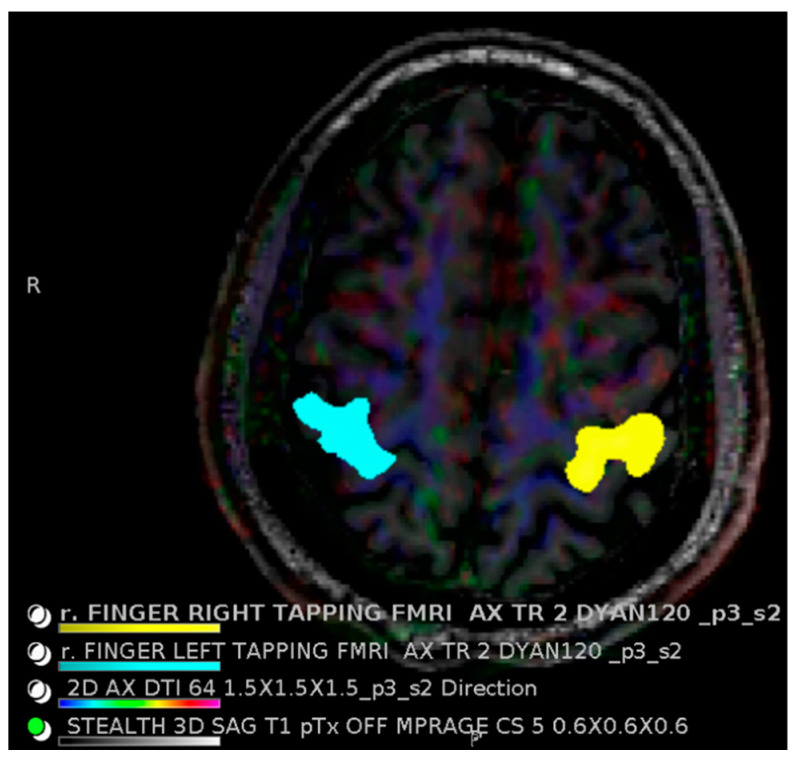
Functional MRI at 7 Tesla provides more than twice the usual BOLD signal and is localized more closely to the center of activation. This healthy volunteer shows finger tapping (left finger in blue and right finger in yellow) localizing to the appropriate anatomic region. Images acquired at the Mayo Clinic.
